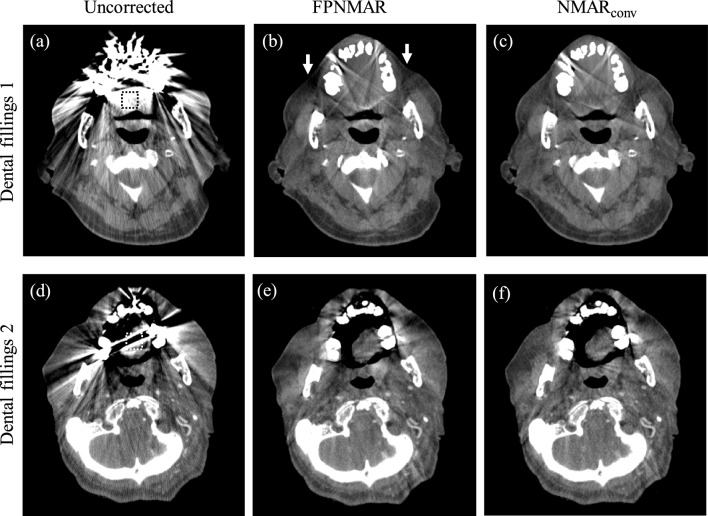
Uncorrected, FPNMAR-processed, and NMAR_conv_-processed images for two cases of dental fillings. Arrows indicate portions with a CT number decrease. The window’s width and level were set at 500 HU and 40 HU. The position of ROI for measuring the AI_n_ is presented with the dashed box in each uncorrected image
